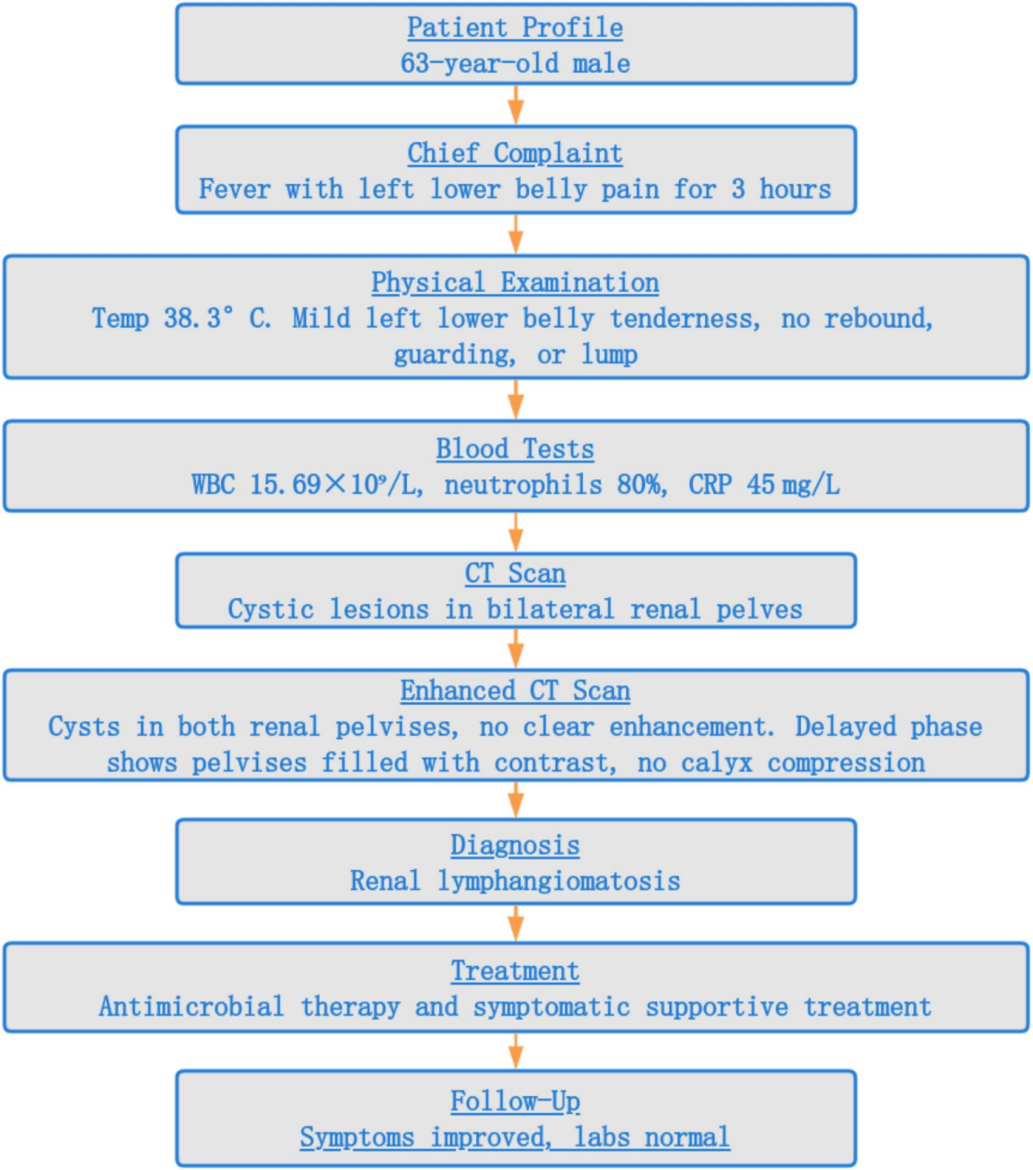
Patient care timeline: profile, chief complaint, examination, investigations, enhanced CT, diagnosis, treatment, and follow-up.
